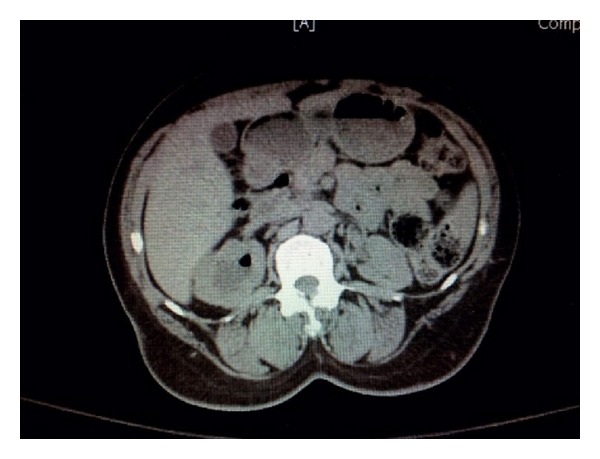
CT scan of the abdomen and pelvis showing right-sided ureteropelvic junction stenosis with moderate to marked hydronephrosis, air in the collecting system and intraparenchymal area, and increased echogenicity of the right renal parenchyma suggestive of parenchymal disease.
